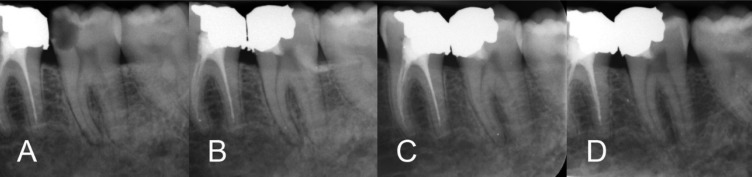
A)* preoperative intraoral x-ray*; B) *immediate postoperative x-ray;* C) *12-month follow-up;* D) *18-month follow-up
